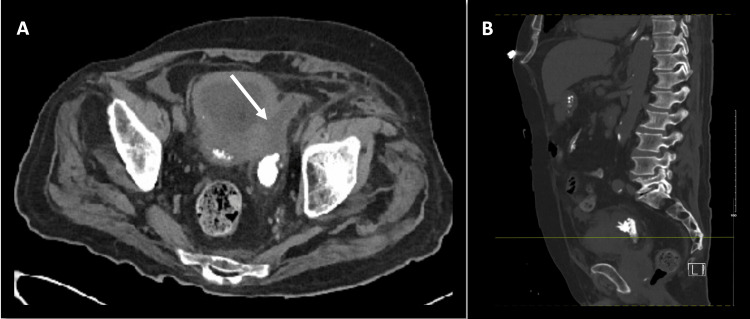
(A) axial and (B) sagittal views of non-contrast CT abdomen in the index patient, showing a thickened vesical bladder wall and large left bladder wall diverticulum (arrow) with intraluminal calculus in place posteriorly (the inferior aspect of the calculus shown in axial view)
